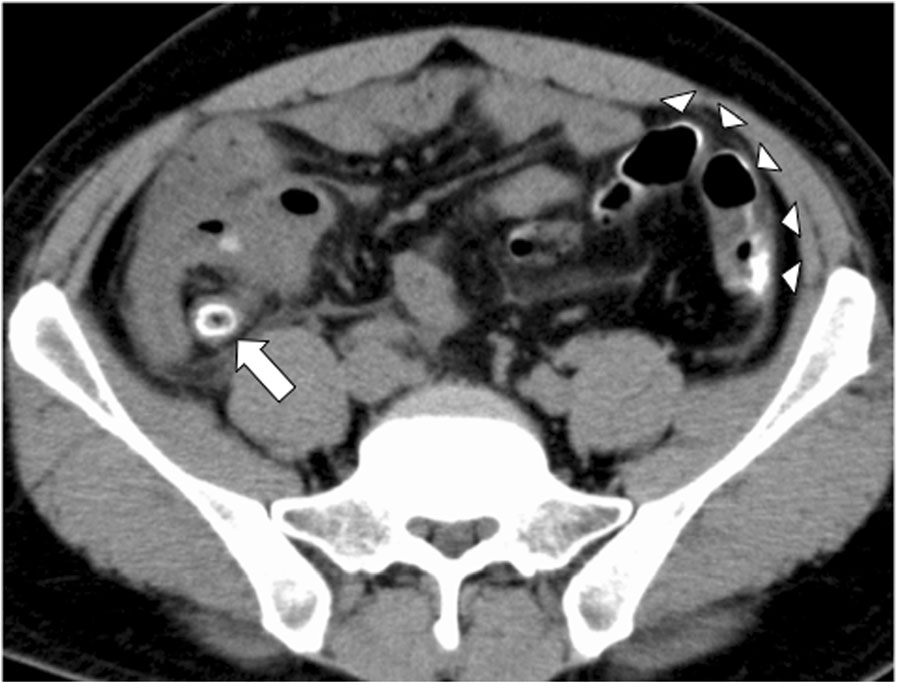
Mural calcification of the appendix and descending colon. Computed tomography showed thickening of the appendix associated with mural calcification and fluid collection (white arrow), and mural calcification of the descending colon to the rectum (white arrowhead). Extensive curvilinear calcification and calcifications resembling tram tracks were found in the colon and the appendix
